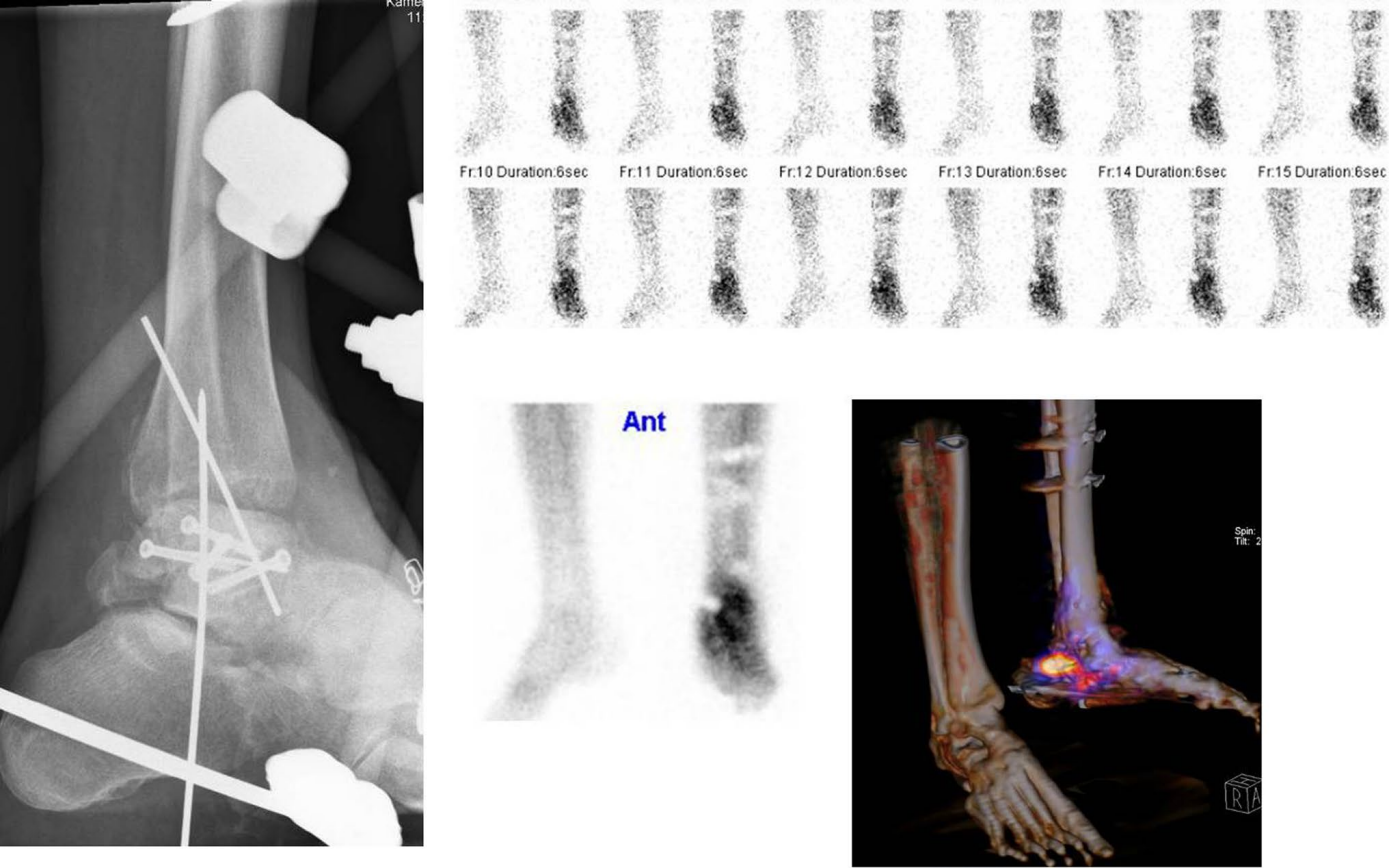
X-ray (*left image*) and bone scan (*upper row* flow phase, *middle image lower row* blood pool phase, *right image lower row* SPECT-CT late phase) of patient B
